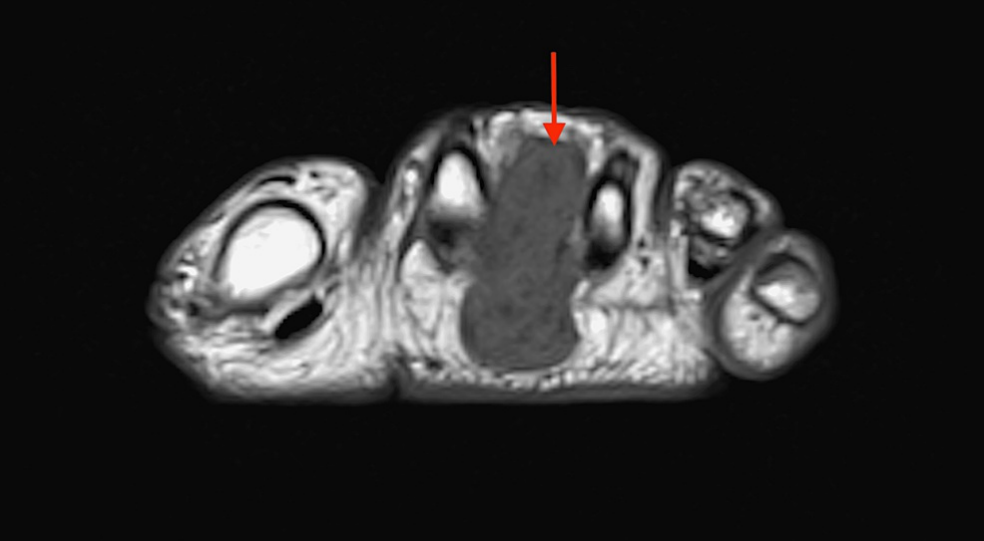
Magnetic resonance imaging displaying a soft-tissue mass with low signal intensity between the second and third metatarsal in a T-1 weighted sequence
